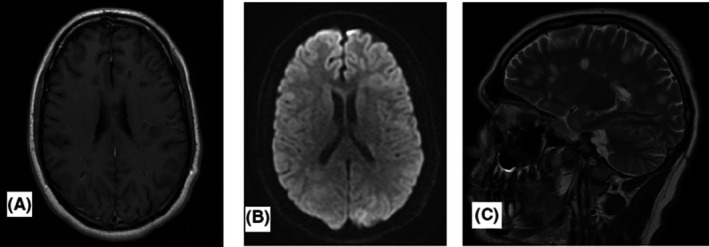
Control scanning axial T1 + control (a) and DWI (b) show the regression of contrast enhancement and high‐signal intensity in general. Sagital T2 (c) shows still multiple MS plaques
